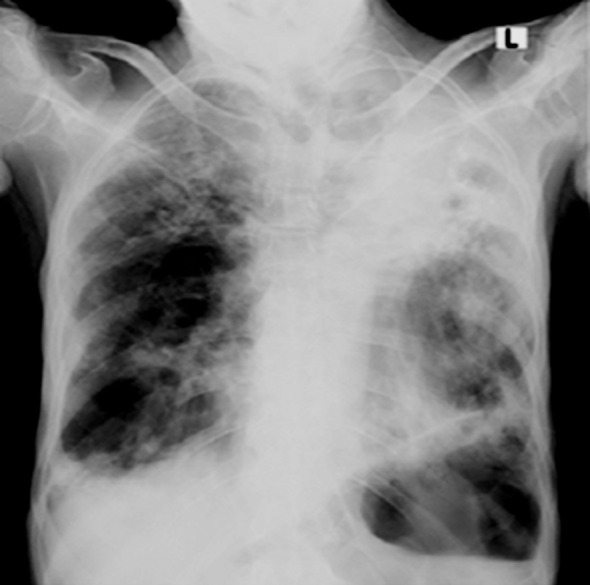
Chest X-ray of a young male patient, having cough, sore throat, rhinitis, shortness of breath and fever but had negative COVID serology. X-ray showed left upper lobe collapse, fibrocalcific changes, atelactatic bands in left lower zone, elevation of ipsilateral hemidiaphragm with few calcific granulomas in right middle zone, these are sequelae of healed pulmonary koch’s. (Consistent with History) In homogenous airspace shadowing in both upper and right mid zones, bilateral pleural effusions suggestive of acute overwhelming infection on background of chronic healed granulomatous disease. Patient was advised for gene expert test to rule out reactivation of pulmonary Koch’s.
